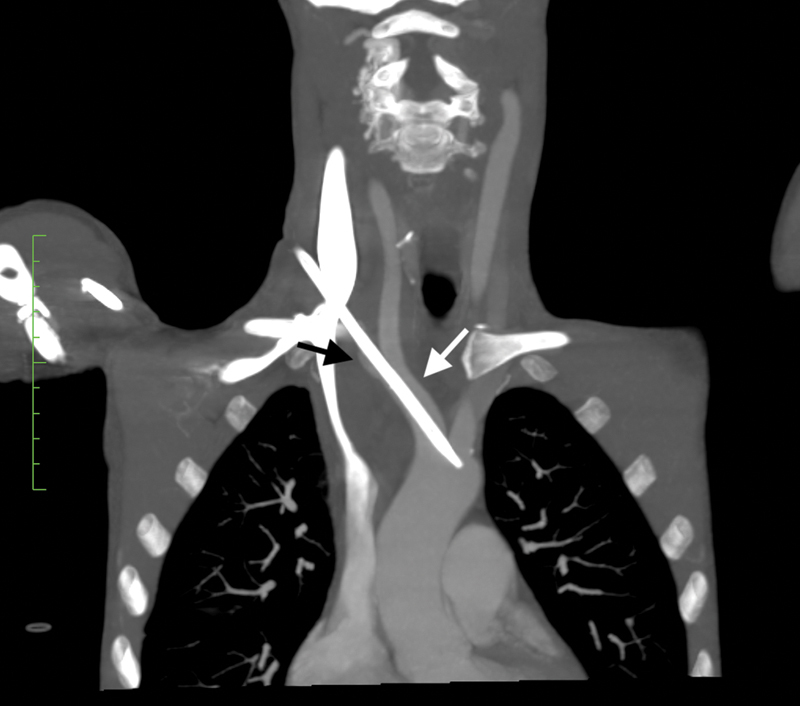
Computed tomography angiography image, in coronal section, showing the course of the malpositioned dialysis catheter, after puncturing through the internal jugular vein (medial aspect). The catheter is seen to course through the right subclavian artery (black arrow), with the tip within the brachiocephalic trunk (white arrow).
